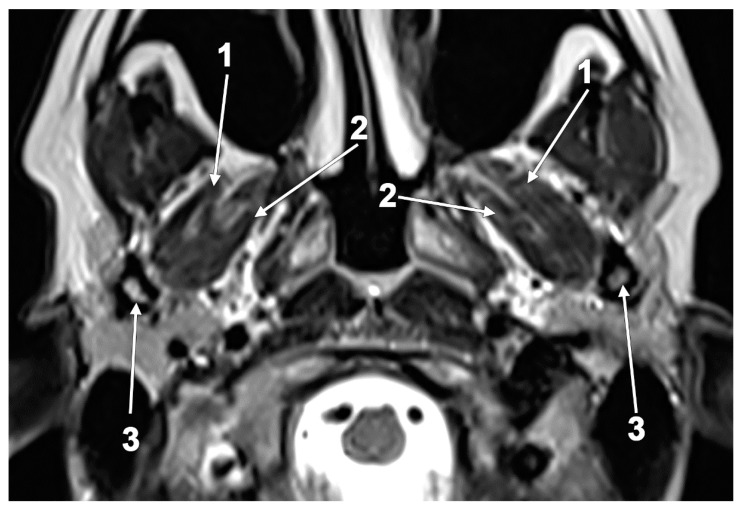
Axial MRI slice through the inferior heads of the lateral pterygoid muscle (ILPMs). Inferior view: 1. lateral bundle of the ILPM; 2. medial bundle of the ILPM; and 3. neck of the mandible.
